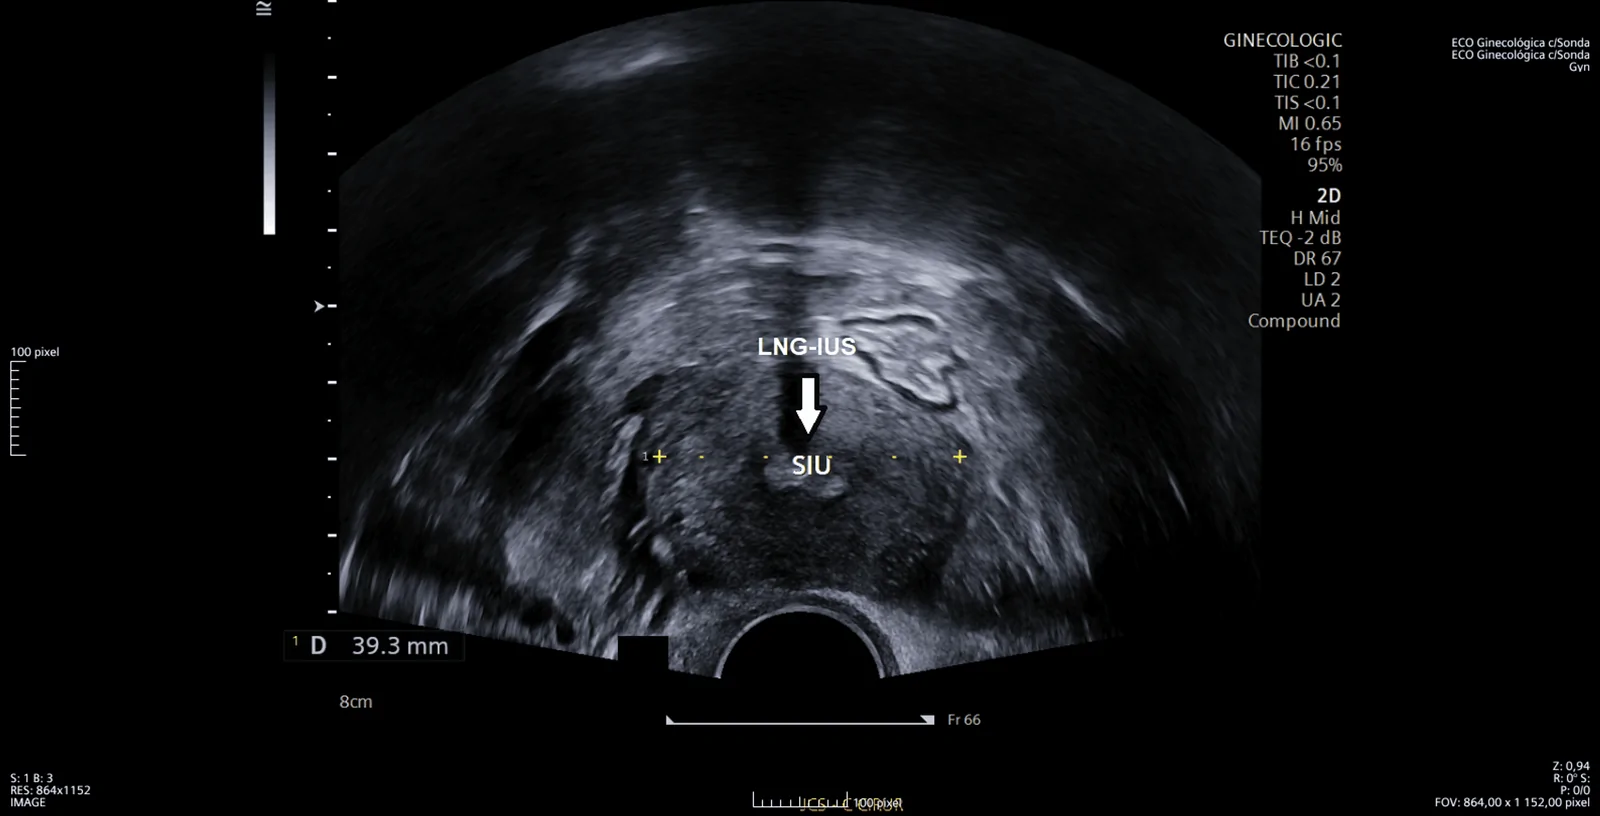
Follow-up TVUS (transverse view) confirming correct fundal positioning of the LNG-IUS. The white arrow indicates the upper end of the LNG-IUS near the uterine fundus. TVUS: Transvaginal ultrasound; LNG-IUS: Levonorgestrel-releasing intrauterine system
